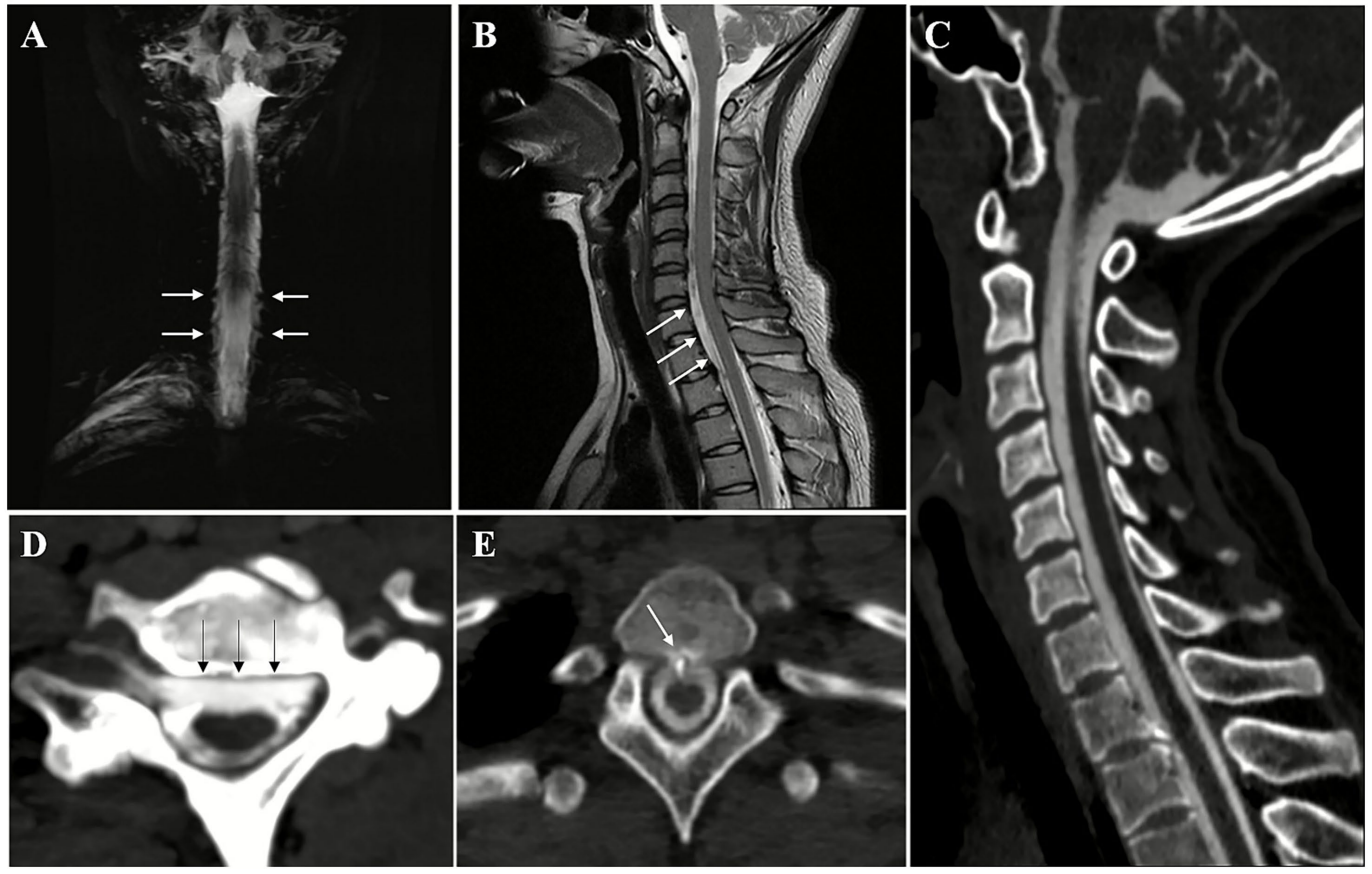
Evaluation of the patient’s spinal cerebrospinal fluid (CSF) leak. Heavily-T2 weighed MR myelography imaging showed increased CSF signal (white arrows) on the external side of the spine at the cervicothoracic junction (A). Whole spine T2 sequence revealed epidural fluid collections (white arrows) in front of the spinal cord at the cervicothoracic junction (B). Sagittal (C) and Axial (D) CT myelography (CTM) showed epidural fluid collections (black arrows) in front of the spinal cord at the cervicothoracic junction and upper thoracic segment. Osteophyte formation at the T1/2 vertebrae, projecting anteriorly toward the spinal cord, was observed, with the osteophyte (white arrows) almost touching the anterior side of the spinal cord, potentially piercing the dura (E). The location of the CSF leak was suspected to be at the T1/2 osteophyte level.
